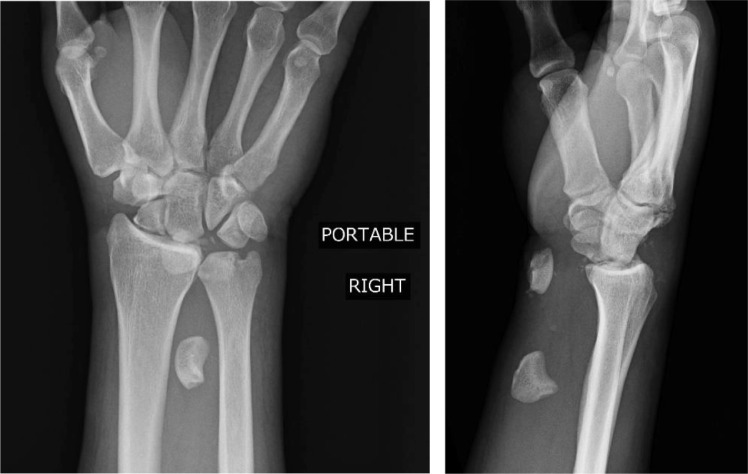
Patient 1: Initial radiographs of the right wrist showing a trans-scaphoid lunate dislocation. There is a fracture of the scaphoid waist with the proximal pole displaced into the volar wrist. There is severe dislocation of the lunate into the distal volar forearm.
